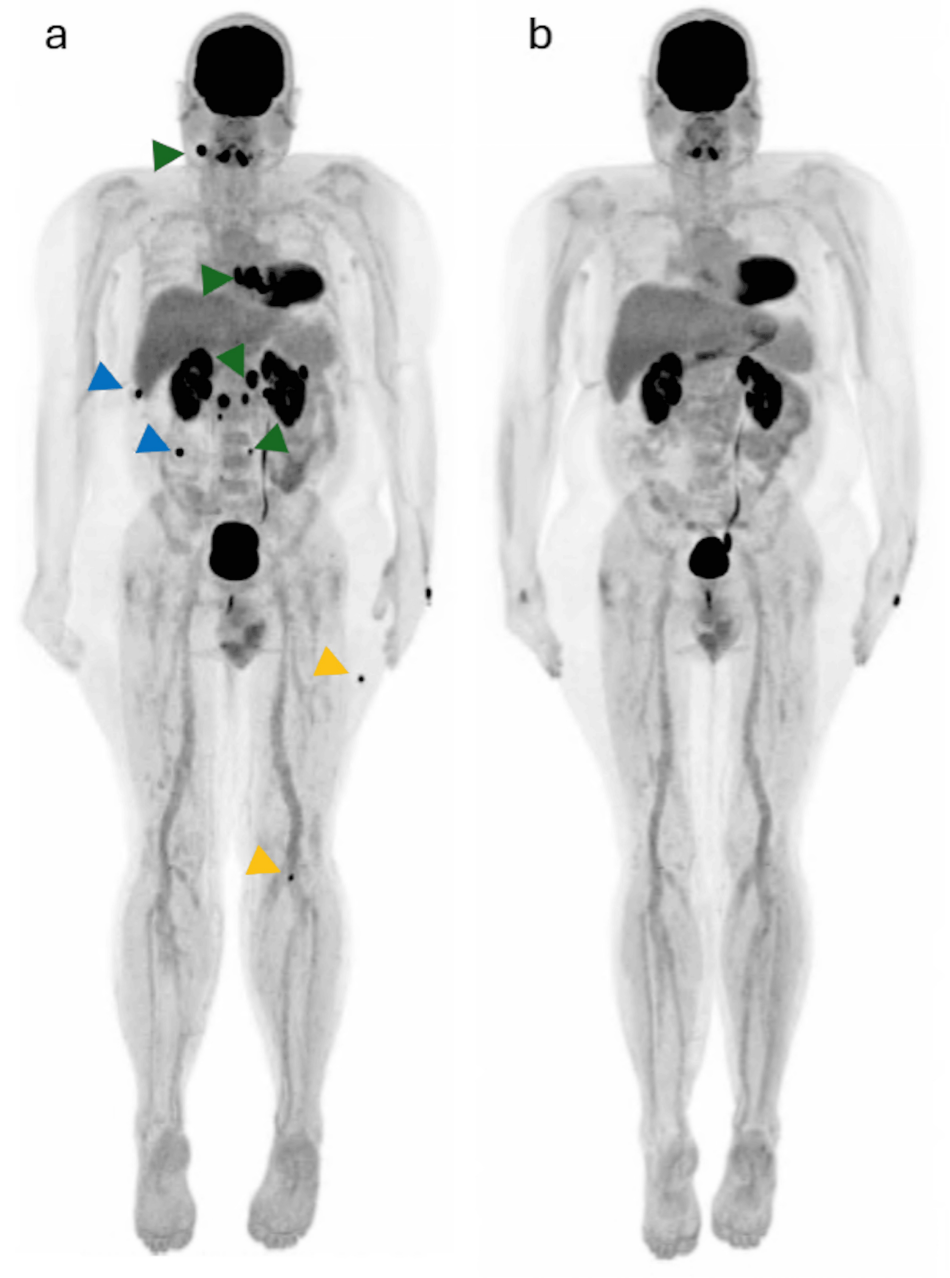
a) Maximum intensity projection (MIP) image demonstrating FDG-avid cervical and retroperitoneal lymph nodes (green arrowheads), peritoneal implants (blue arrowheads), and muscle lesions (yellow arrowheads). b) Follow-up MIP image obtained three months after the initial FDG-PET/CT, showing a marked reduction in FDG uptake with a near-complete metabolic response.
